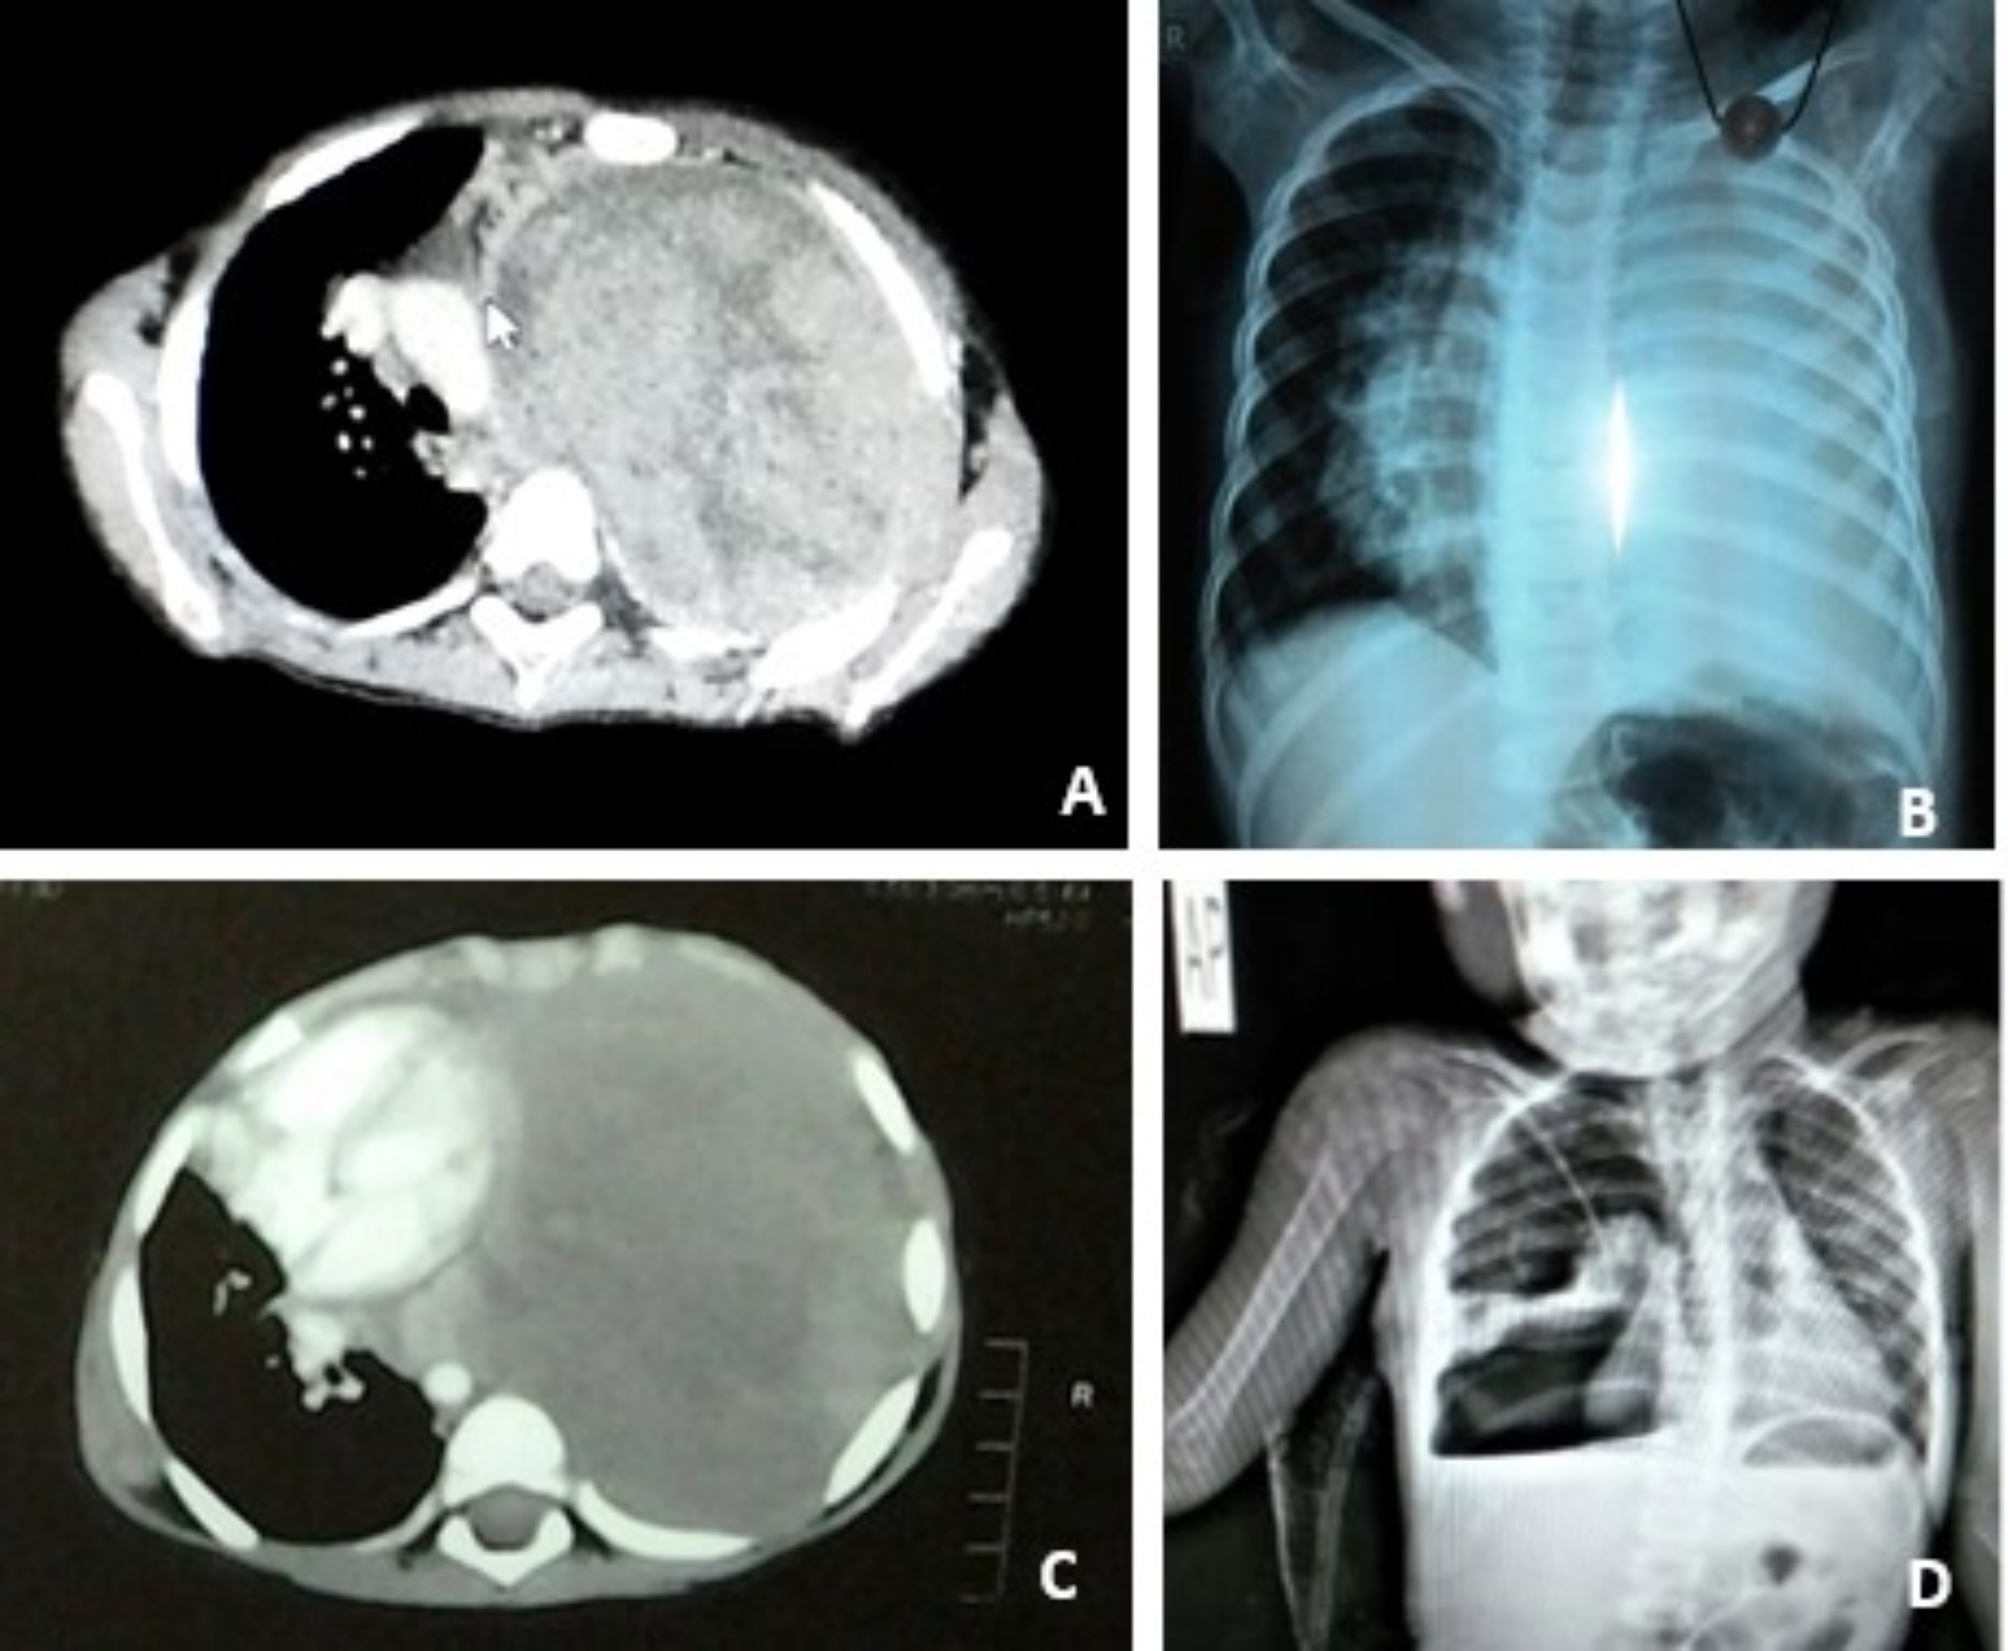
Sample of radiologic findings in the included patients. (A) A large sized heterogenous mass on the left side (Case 2). (B) Opacification of the left hemithorax (Case 3). (C) CT showing homogenous mediastinal mass (Case 3). (D) PXR showing hydro-pneumothorax on the right side (Case 4)
